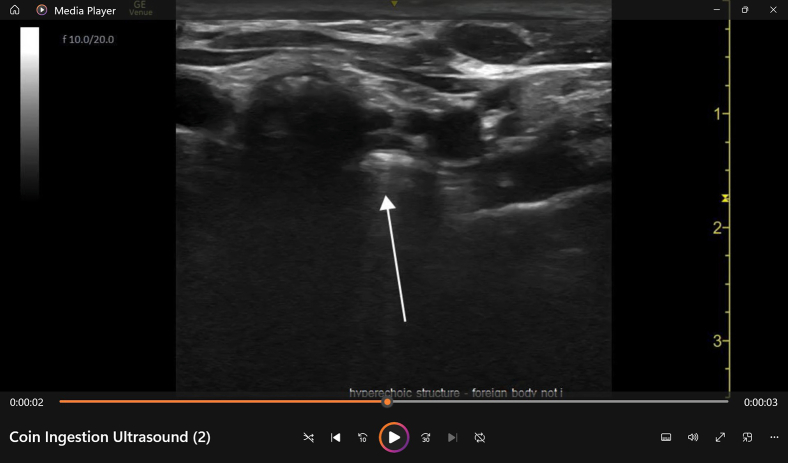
Transverse neck ultrasound demonstrates a hyperechoic foreign body with posterior acoustic shadowing within the esophagus, posterior to the trachea with a labeled arrow.
